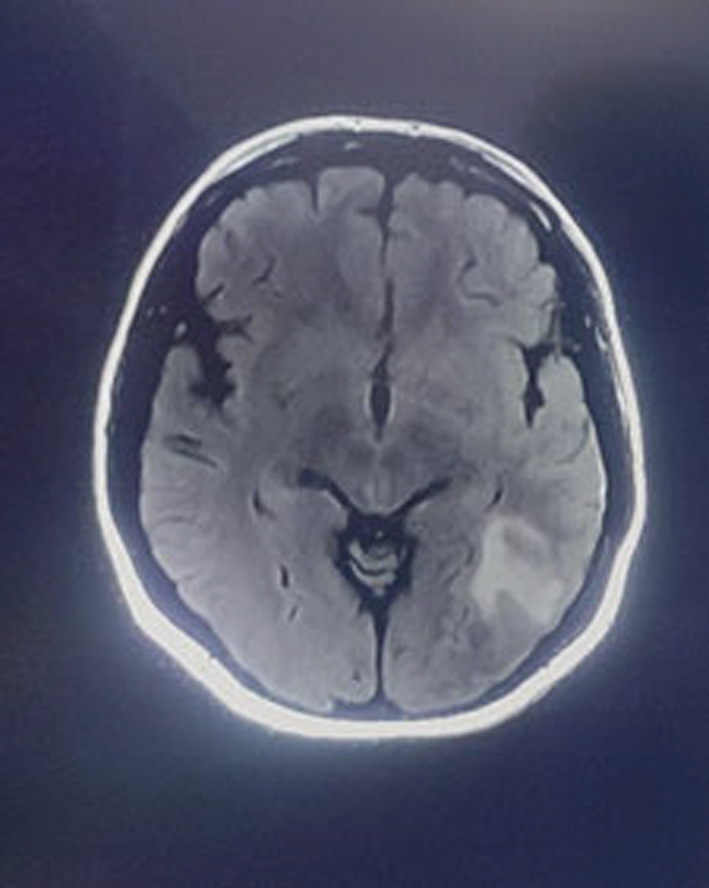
Axial brain CT scan showed hyperdensity in occipital lobe in favor of parenchymal bleeding.
